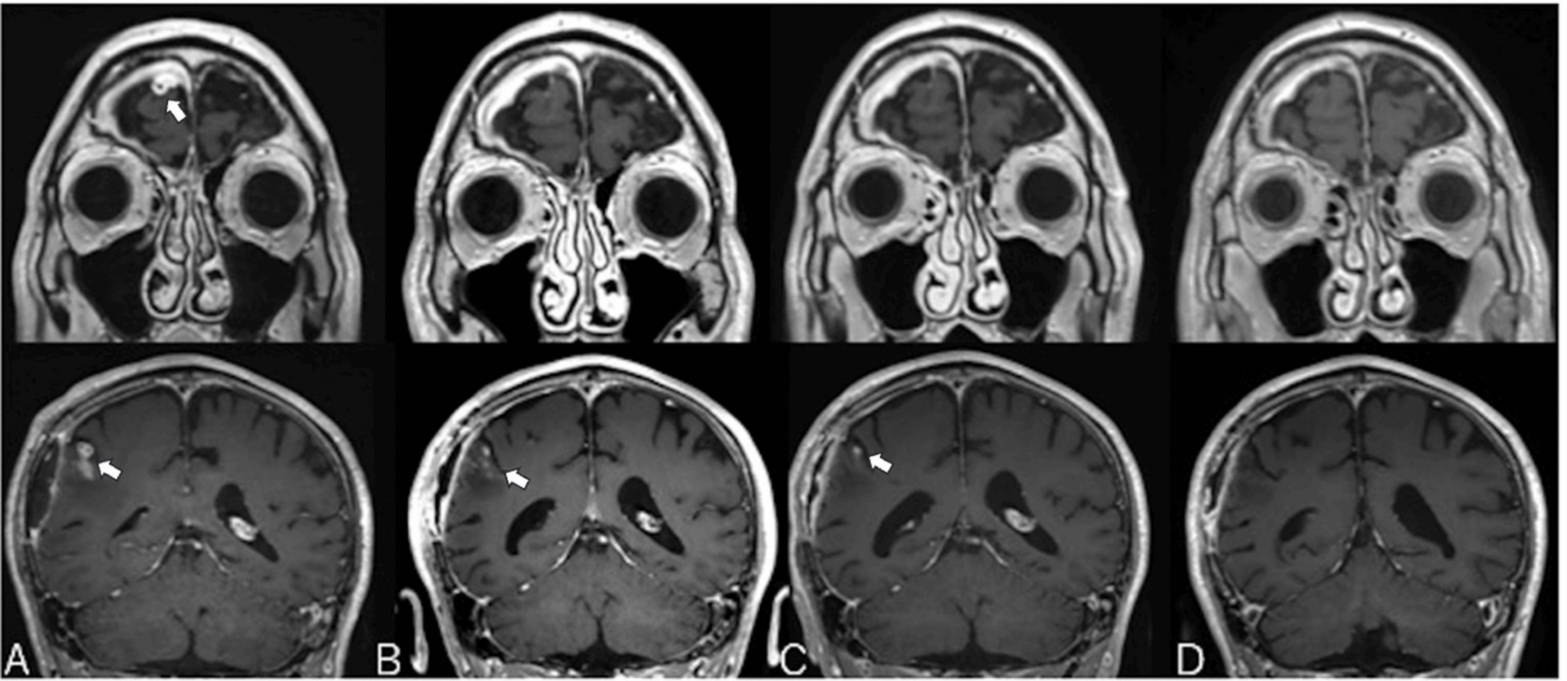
Coronal view of contrast-enhanced magnetic resonance image. (A) At six weeks after antifungal therapy initiation: shrinkage of the right temporal lobe lesion (arrow in the lower image), but a new lesion was detected in the right frontal lobe (arrow in the upper image). (B) At 12 weeks after antifungal therapy initiation: further reduction of the right temporal (arrow in the lower image) and frontal lobe lesions. (C) At two weeks after shifting to fluconazole (pre-discharge): further reduction of the right temporal (arrow in the lower image) and frontal lobe lesions. (D) At five weeks after shifting to fluconazole (post-discharge): absence of contrast-enhancing lesions in the right temporal and frontal lobes.
